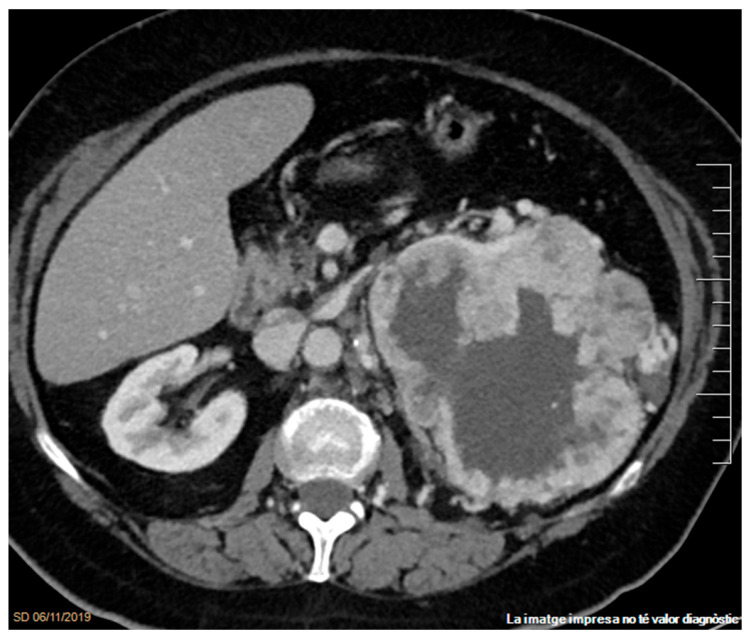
Infiltrating left clear cell RCC with necrosis. Contrast-enhanced axial CT image in the venous phase demonstrates a hypervascular mass with heterogeneous enhancement, attributable to a large non-enhancing necrotic area. Peritumoral vessels are visible within the perirenal space.
